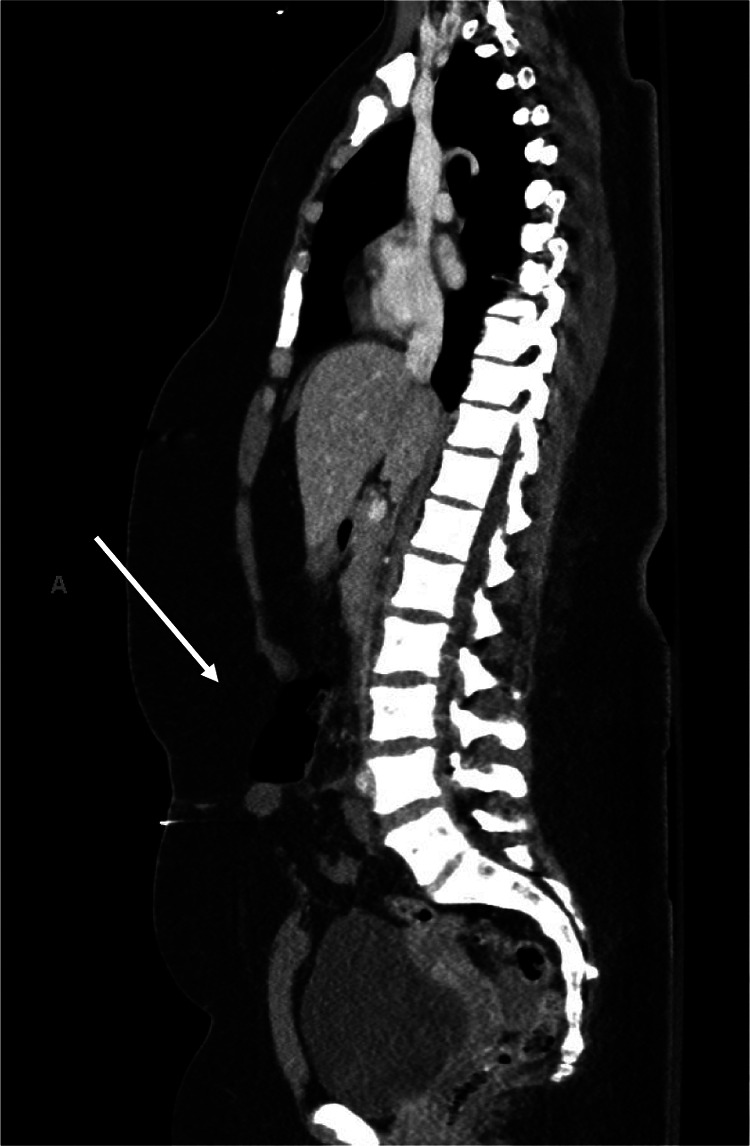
Sagittal computed tomography showing rectus muscle disruption with abdominal content herniation (arrow).
